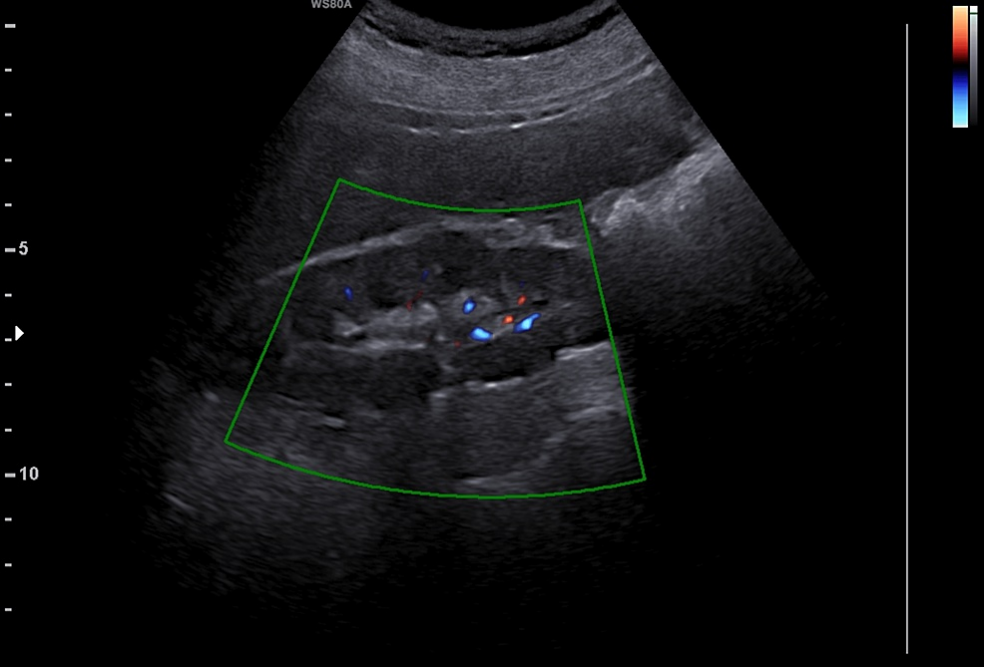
Postoperative ultrasound imaging of the right kidney (our case) The absence of the preoperative dilatation of the pelvicalyceal system and the presence of adequate perfusion of the renal parenchyma is evident.
